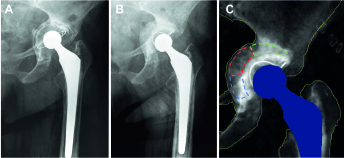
A: Example of an anterior–posterior (AP) hip radiograph with preoperative osteolytic bone deterioration with excessive cup protrusion and loosening. B: Postoperative AP radiograph with reconstruction of the contained acetabular bony defect using the bone impaction grafting technique without metal meshes. C: With dual-energy X-ray absorptiometry, bone mineral density was measured in 3 separate regions of interest covering the postoperative acetabular impacted bone graft: cranial (green), medial (red), and caudal (blue) to the polyethylene cup. The same ROI template was used for each subsequent time interval.
